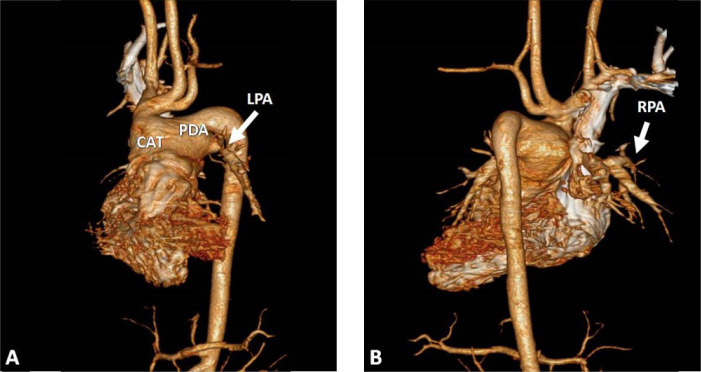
Three-dimensional volume-rendering computed tomography angiography:
